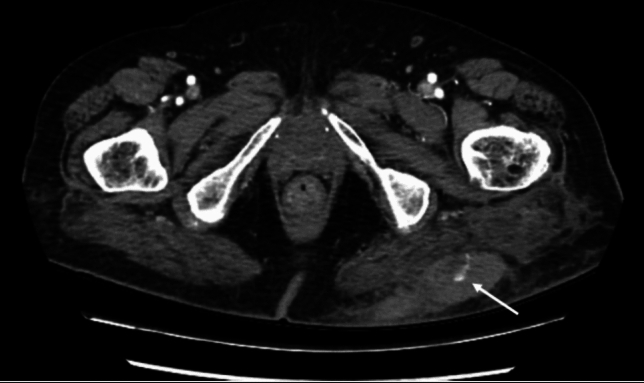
96-year-old female presenting to the emergency department after a fall from standing height. Clinically she had a large left buttock haematoma. Dual bolus CT scan demonstrating a large left gluteal subcutaneous haematoma with contrast extravasation. The patient was successfully managed conservatively
